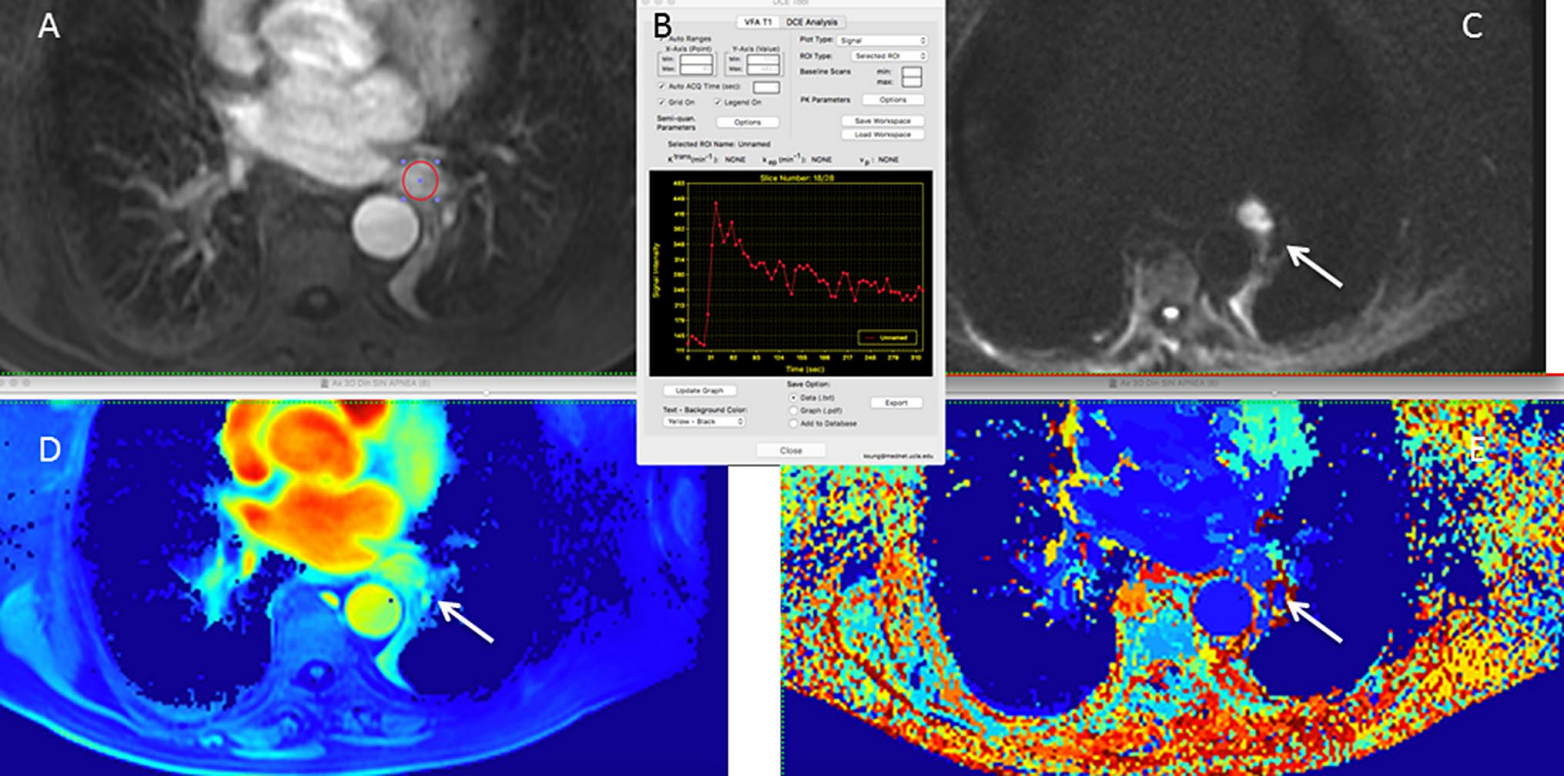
Evaluation of angiogenesis of NSCLC with perfusion MR (same patient of Fig. 2). Dynamic contrast-enhanced MR sequences. a Dynamic-enhanced postcontrast image shows a left hilar mass (ROI), which demonstrated in (b) the signal-intensity-time curve a fast uptake of contrast and delayed wash-out suggesting is malignant origin, as confirmed in (c) high b-value DWI where the lesions shows high signal intensity (arrow). d, e Semiquantitative perfusion maps of peak time and area under the curve better depicts the vascular characteristics of the tumor (arrows)
